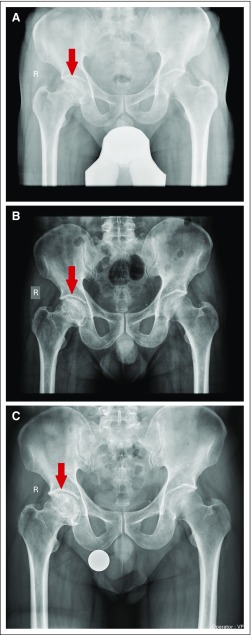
(A) Radiograph of a 22-year-old patient at the start of therapy showing Ficat-Arlet stage II avascular necrosis of right femoral head. (B) Follow-up radiograph at 1 year showing no progression. (C) Pelvis radiograph at 7 years showing no progression/collapse of the femoral head and good radiologic outcome.
